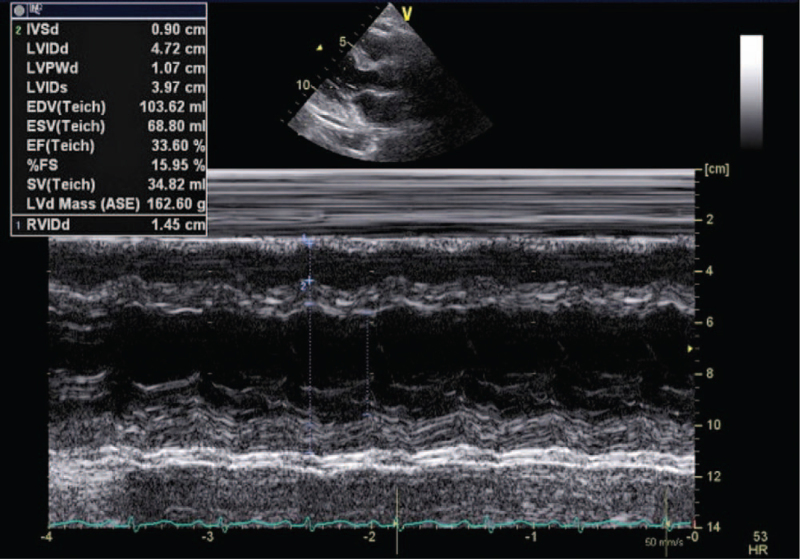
M-mode echocardiography demonstrating low left ventricular ejection fraction.
